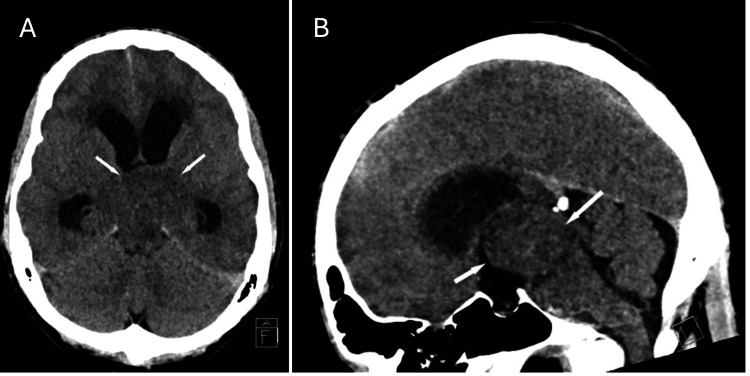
Non-contrast CT at admission (A) Axial and (B) sagittal images. Arrows indicate a mass isodense to white matter filling the third ventricle, with associated obstructive hydrocephalus. CT: computed tomography
